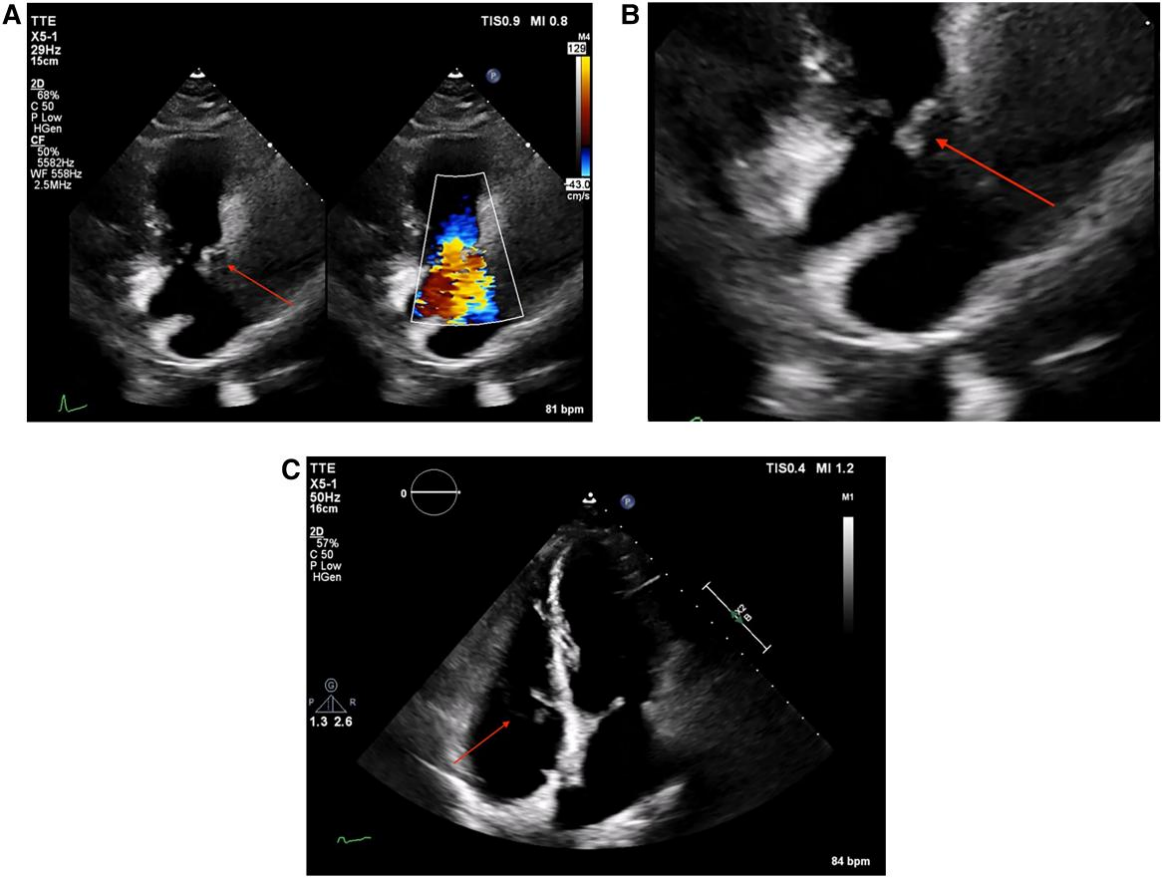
(*A–C*) Transthoracic echocardiogram showing severe tricuspid regurgitation due to the rupture of the anterior papillary muscle. Arrows indicating the flail leaflet.
